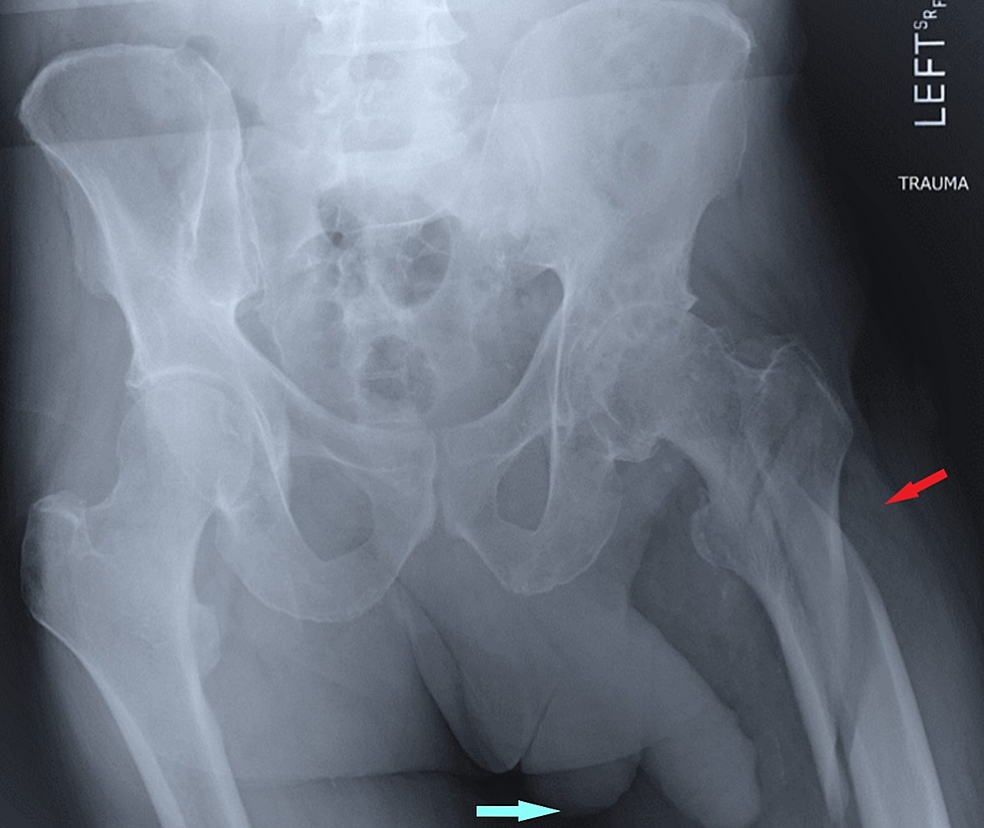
Anteroposterior radiograph of the pelvis demonstrating left-sided pelvic shadowing to the ipsilateral peri-trochanteric hip fracture. The red arrow is pointing to the fracture. The blue arrow is pointing in the direction of the pelvic shadowing towards the side of the fracture.
